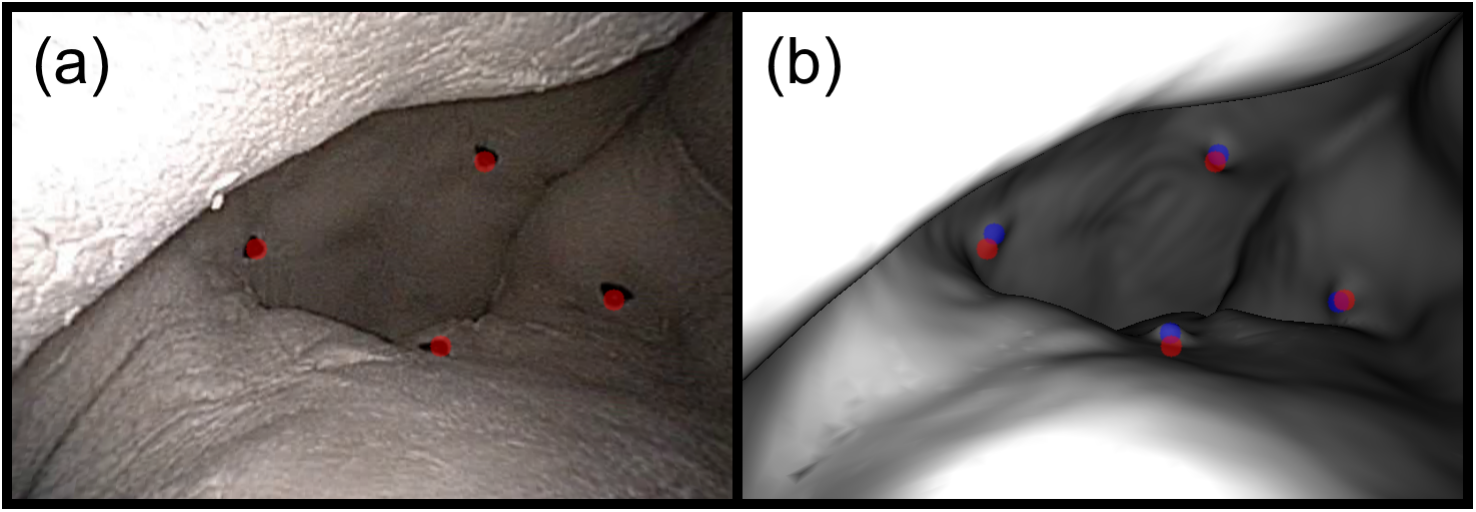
The measurement of CT-space marker positions. (a) A video frame with four markers visible. Their manually-selected pixel locations are overlaid in red. (b) The corresponding virtual endoscopic image rendered at the registered coordinates. The manually-selected pixel locations are overlaid in red and the image-plane projections of their true 3D locations are overlaid in blue. The average 3D error for these four measurements was 0.29 cm, and the average 2D error was 11 pixels.
